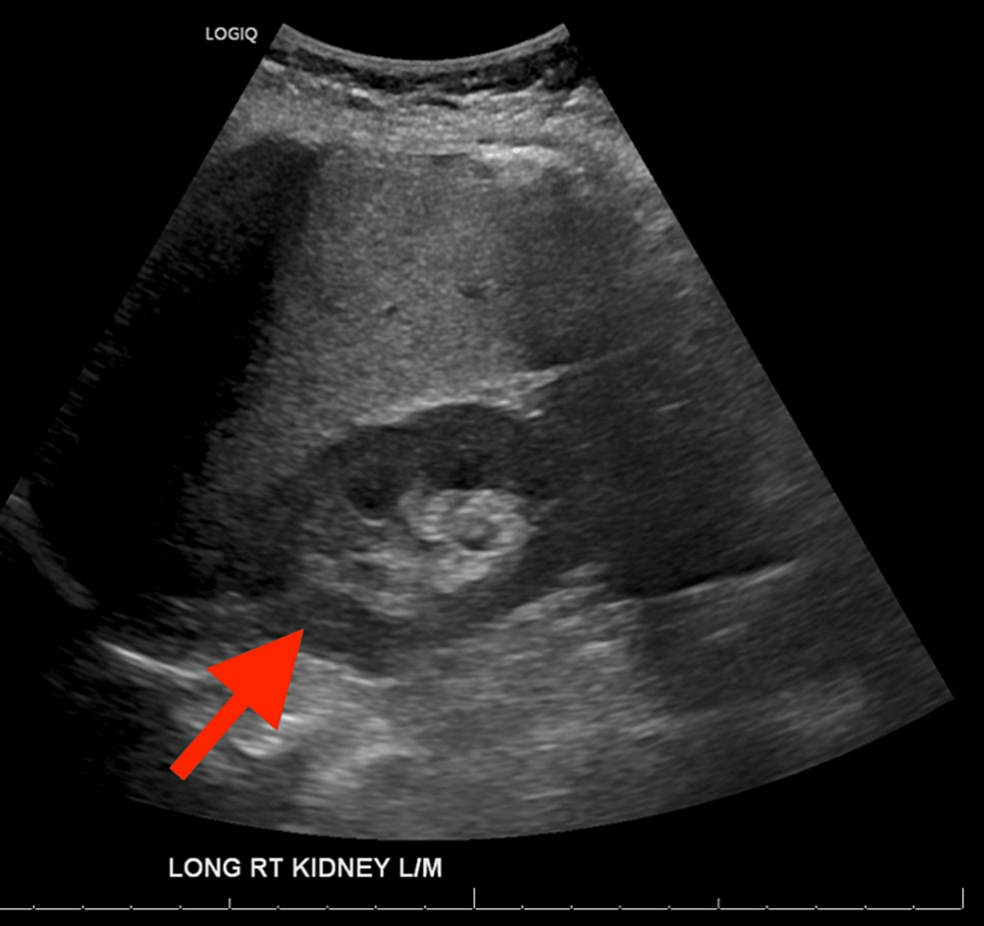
Right upper quadrant ultrasound demonstrating hydronephrosis (red arrow)
